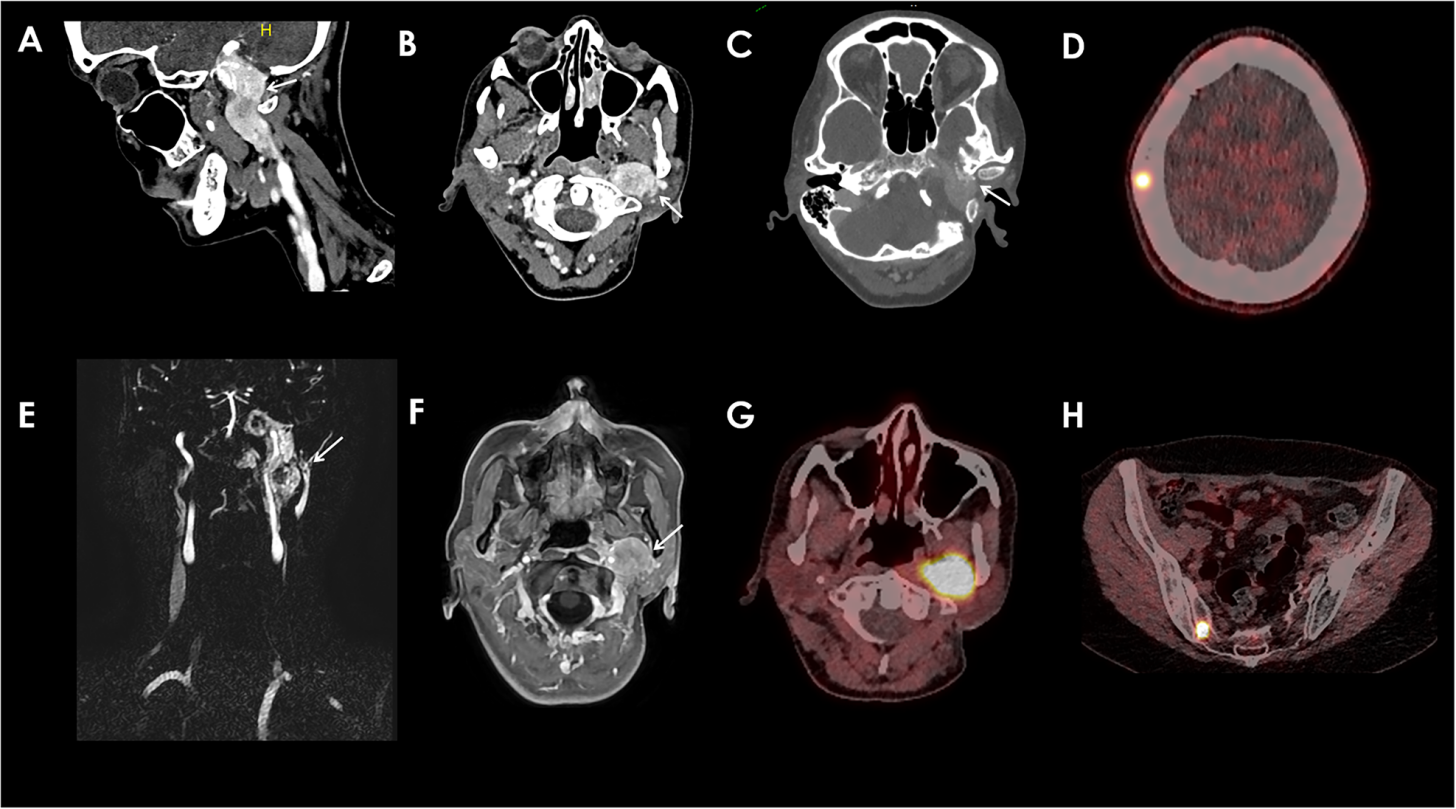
Jugulo-tympanic PGL. Lobulated mass located in the jugulo-tympanic space extending into the jugulo-carotid space, presenting a heterogeneous enhancement on the axial (B) and sagittal CT-Scans (A), measuring 42 × 52 mm, invading the hypoglossal canal, with an adjacent clivus lytic lesion (C). T1 Post Gadolinium Injection axial (F) and Tricks MRA sequence revealing the hypervascular aspect of the PGL (E). Increased 58 MBq de ^68^GA-DOTATOC uptake of the mass on the PET-CT (G) (SUVmax = 33,8). Lytic bone lesion of the skull (D) and the right iliac bone (H) with increased uptake on PET-CT (SUVmax = 41,34)
